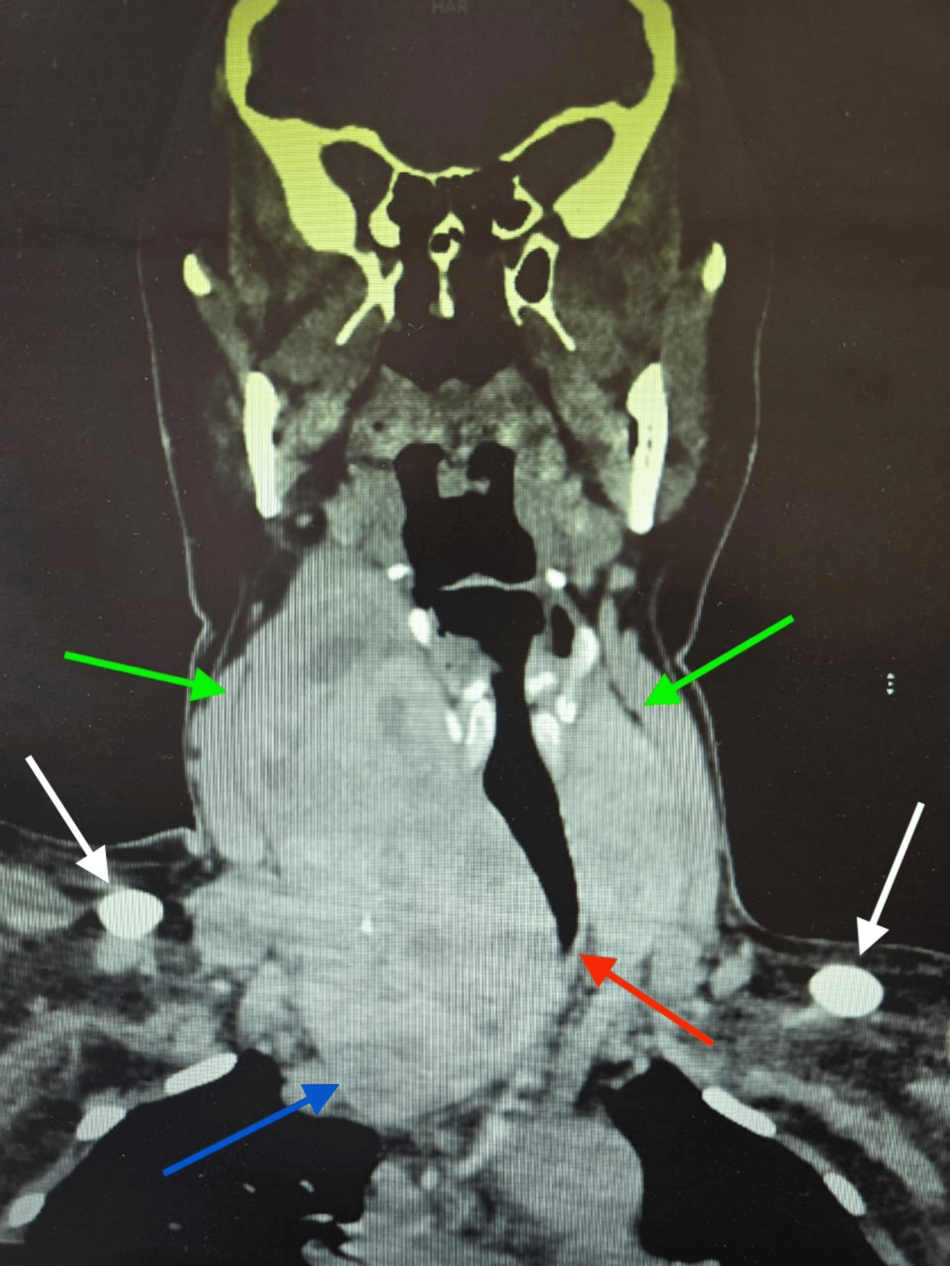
Coronal CT image of the neck without contrast demonstrating a markedly enlarged thyroid gland with inferior retrosternal extension reaching just above the confluence of the brachiocephalic veins, without significant compression of these vessels (blue arrow). The common carotid arteries are indicated (green arrows). Leftward tracheal deviation caused by the massively enlarged right thyroid lobe is noted (red arrow). The clavicles are identified (white arrows). Despite tracheal deviation, no significant airway compromise is evident on imaging.
